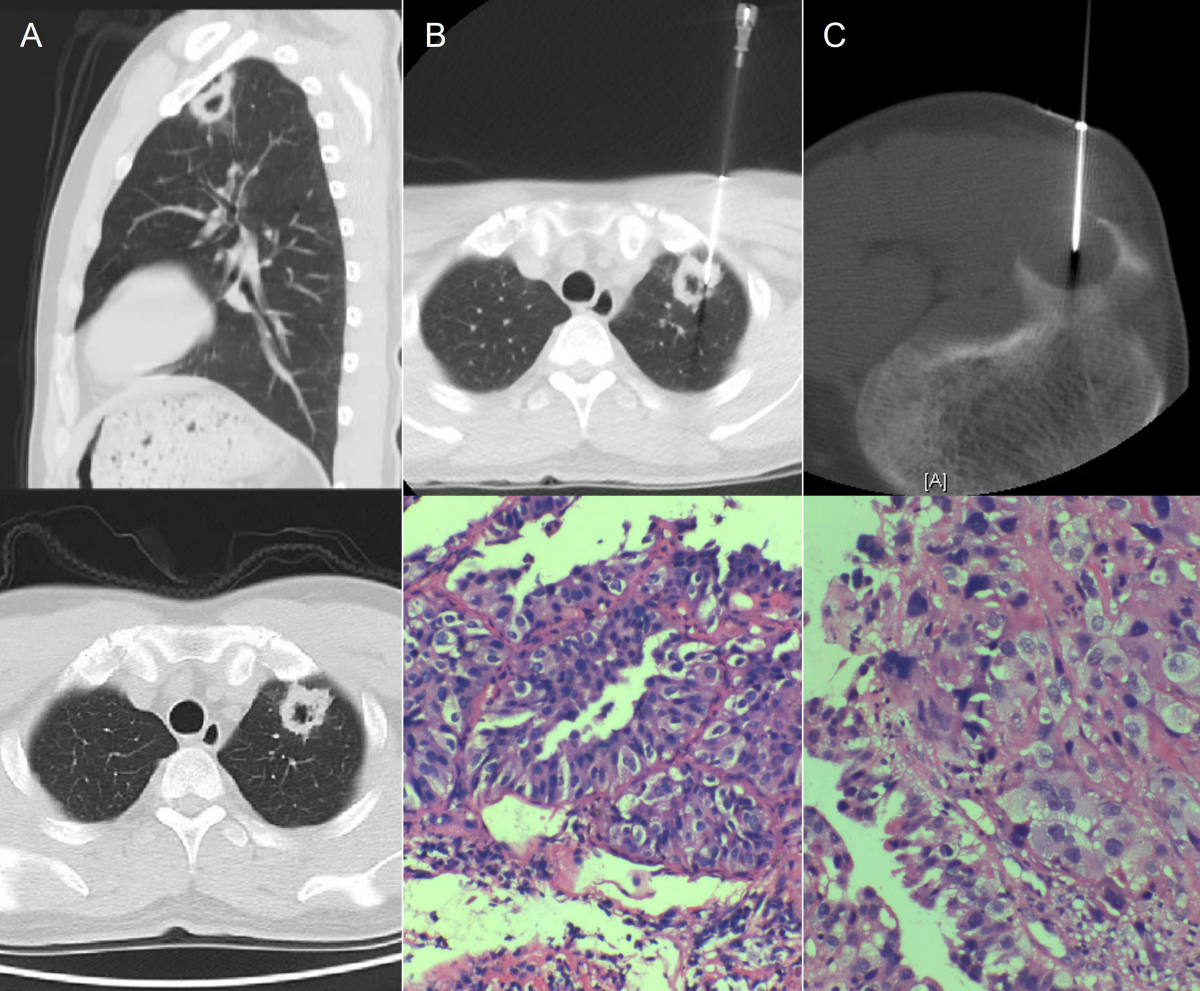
(A) Contrast-enhanced CT scan revealed an irregular mass measuring (28mm×26mm×23mm) in the posterior apical segment of the left upper lobe of the lung. CT, computed tomography. (B) CT-guided puncture and pathologic biopsy of the right lung nodule suggested primary adenocarcinoma of the left lung. CT, computed tomography. (C) CT-guided puncture and pathologic biopsy of the right fibular head mass suggested metastatic adenocarcinoma of the right fibula, possibly originating from the lung. CT, computed tomography.
